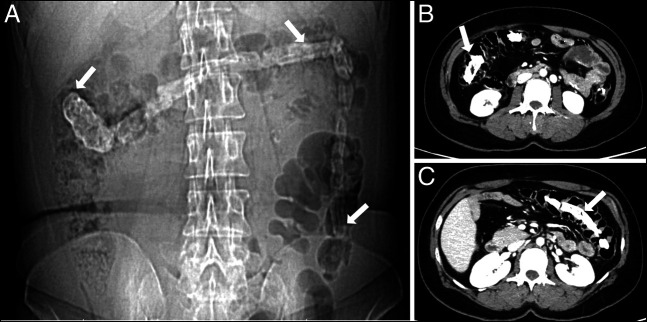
Radiological appearance of gossypiboma. (A) Retained surgical sponge extending from right colon into the transverse colon and up to descending colon (white arrows). (B, C) Abdominal computed tomography scan (axial section) showing gossypiboma within the large bowel loops (white arrows).
